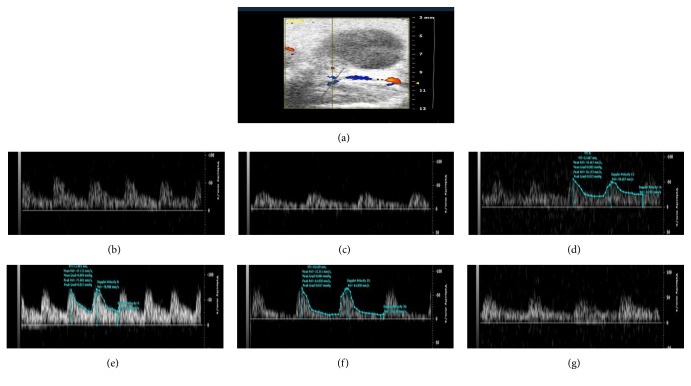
Representative Doppler waveforms of the internal iliac artery. (a) The image of measurement of the internal iliac artery in color mode; (b) the control group; (c) the model group; (d) the aspirin group; (e) the DZD-H group; (f) the DZD-M group; (g) the DZD-L group.
